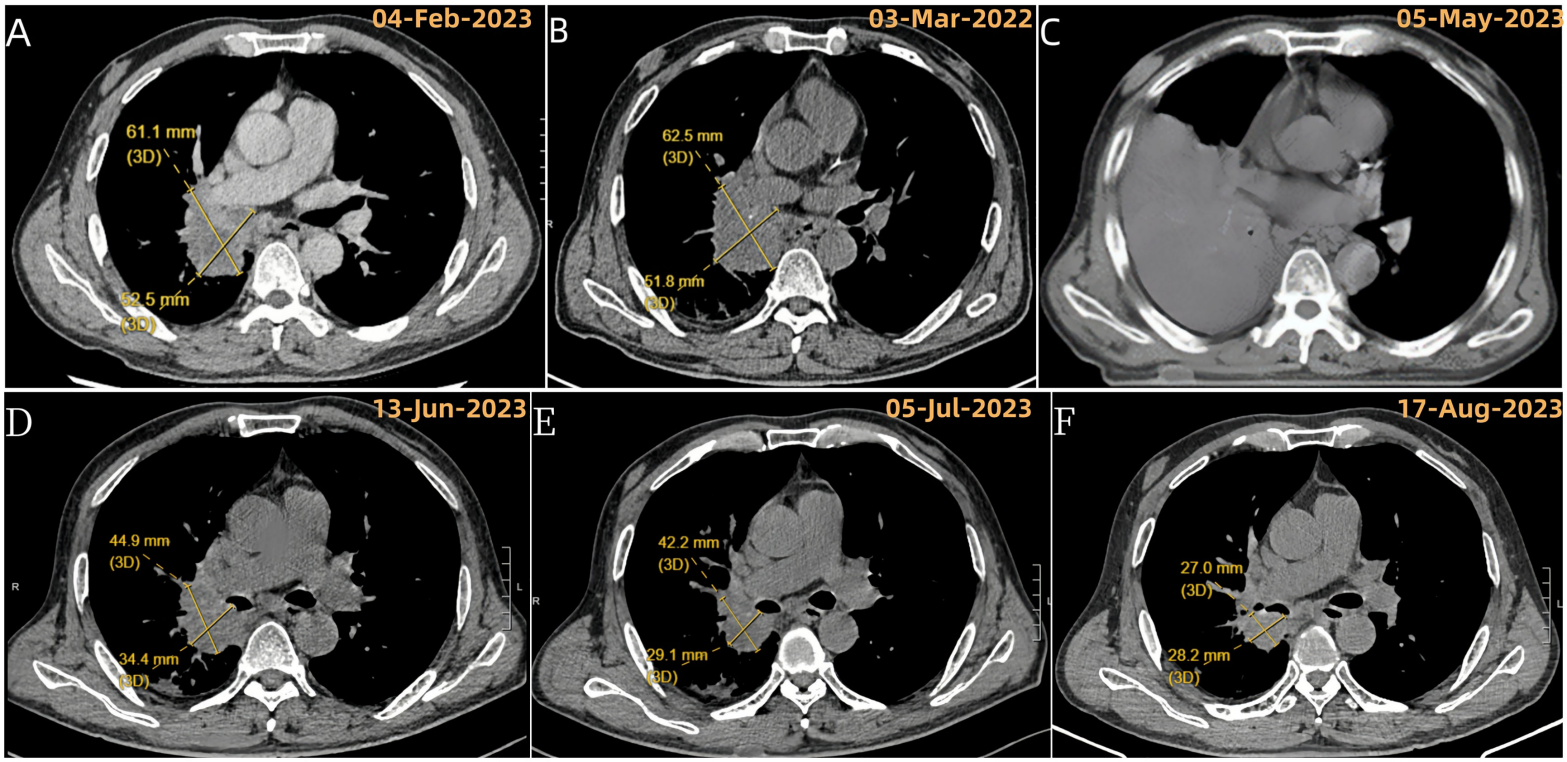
Evolution of right lung target lesion on chest CT. (A) Chest enhanced CT at initial presentation (baseline). (B) After 2 cycles of chemotherapy; (C) After the last tumor resection, before starting second-line treatment (tislelizumab + anlotinib) due to recurrence of atelectasis and inability to measure the target lesion; (D) Following the first cycle of tislelizumab + anlotinib; (E) After 2 cycles of tislelizumab + anlotinib; (F) After 4 cycles of tislelizumab + anlotinib.
